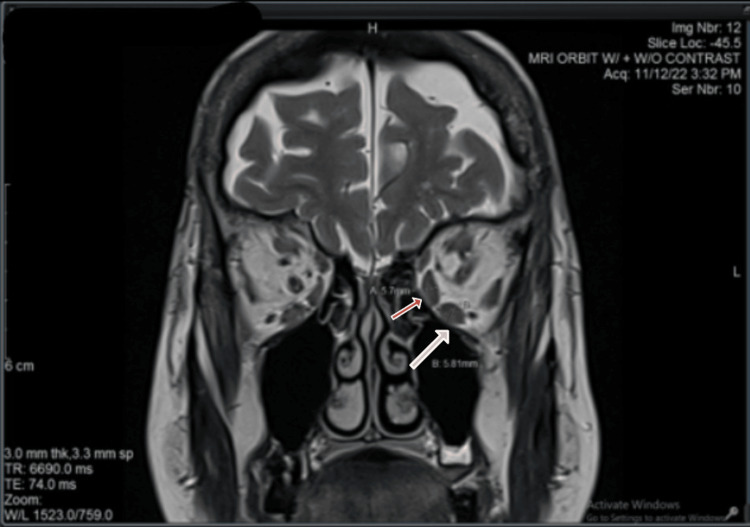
Coronal MRI scan of the extraocular muscles Enlarged left medial and inferior recti muscles measuring at 5.70 mm and 5.81 mm, respectively.
